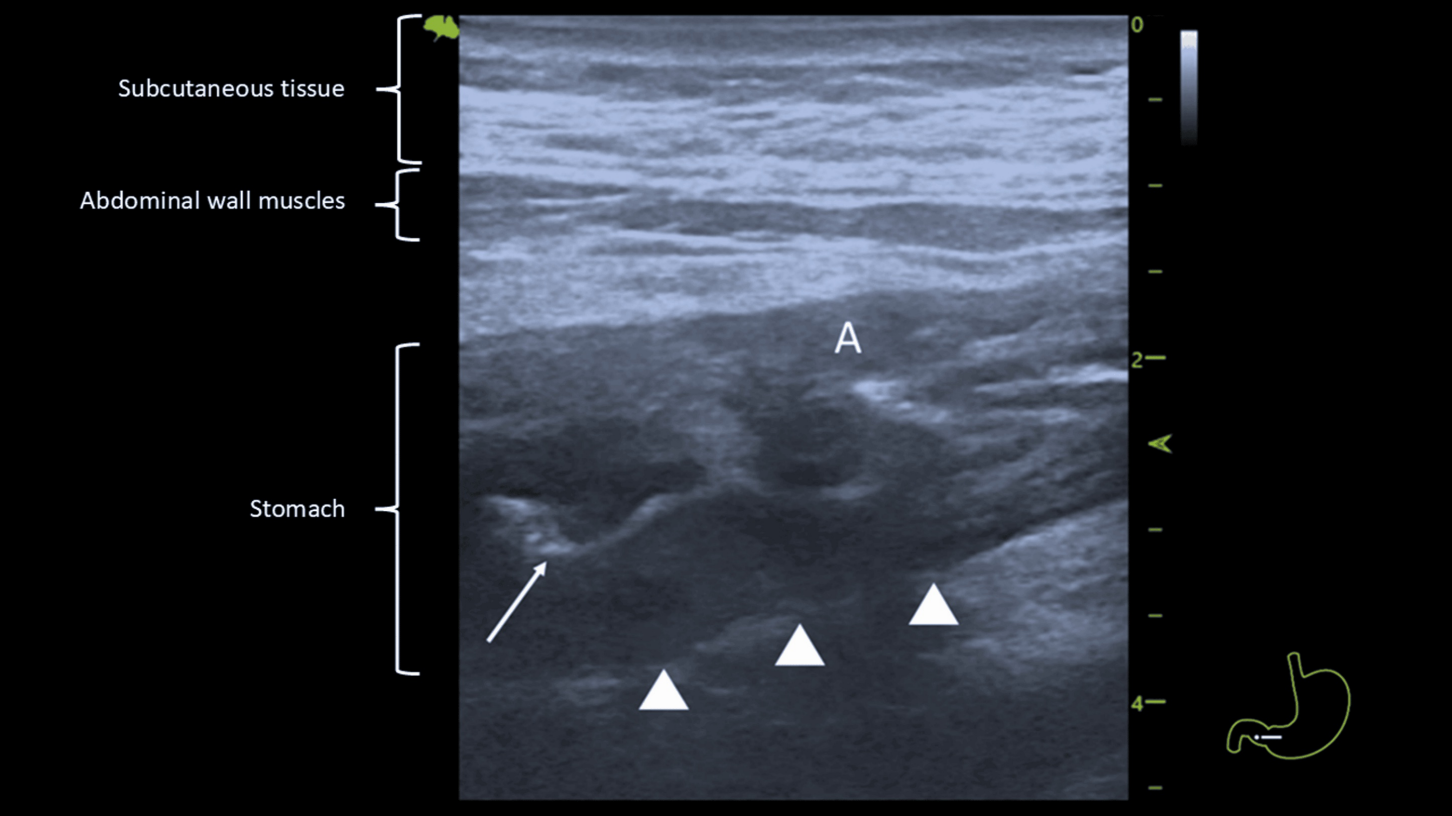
POCUS with a linear probe (8.5 MHz) showing mucosal layer with irregular posterior outer border (arrowheads) and compromised lumen (white arrow). POCUS: point-of-care ultrasound, A: antrum.
